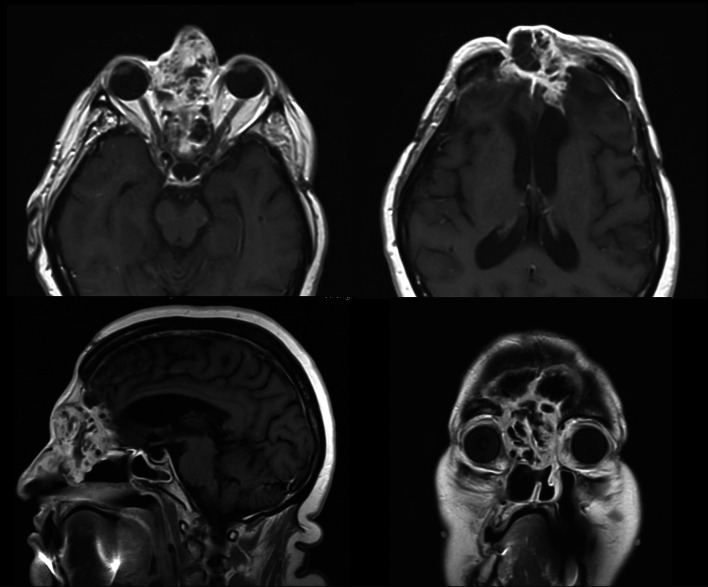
MRI images in axial, sagittal and coronal views showing intracranial infiltration of the AMBL
